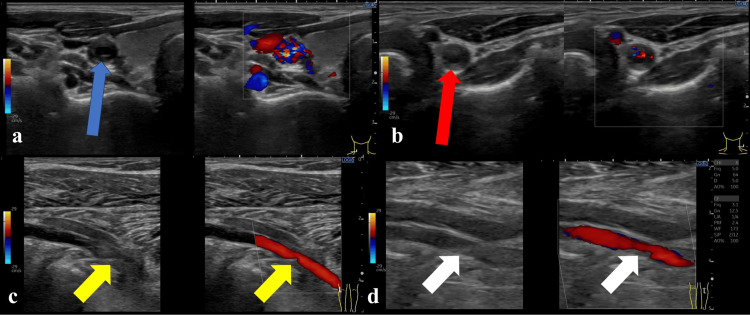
Ultrasonographic findings of the carotid and popliteal arteries a: Ultrasonographic image of the right CCA. The "macaroni sign," characterized by diffuse and circumferential vessel wall thickening, is clearly observed (blue arrow). b: Ultrasonographic image of the left CCA. Similar to the right side, the "macaroni sign" (circumferential vessel wall thickening) is observed (red arrow). c: Ultrasonographic image of the right popliteal artery. Significant vessel wall thickening and luminal narrowing are observed (yellow arrow), suggesting peripheral arterial involvement and potential disease progression. d: Ultrasonographic image of the left popliteal artery. Similar to the right side, wall thickening and narrowing are observed (white arrow), further supporting the suspicion of disease progression toward Numano type V [6]. CCA, common carotid artery
